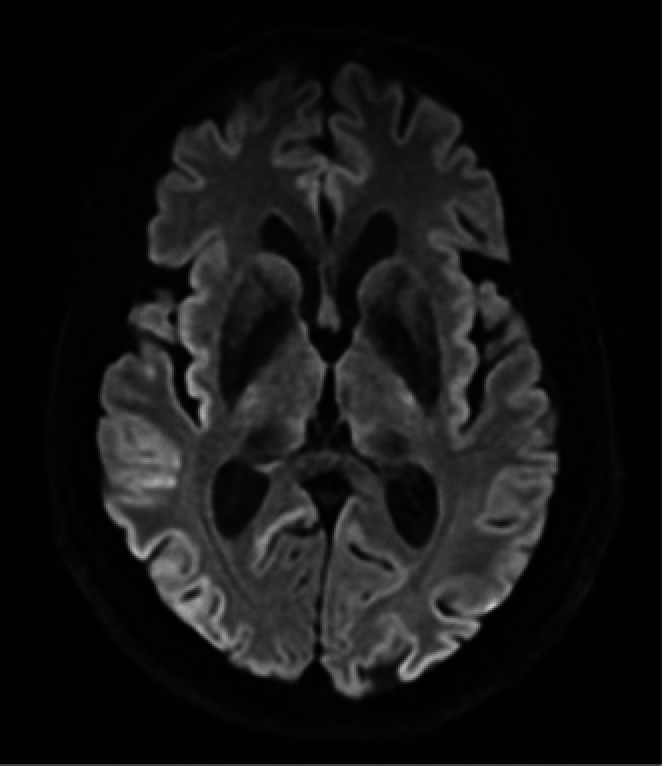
FIGURE 2 MRI with restriction on diffusion (second patient).
